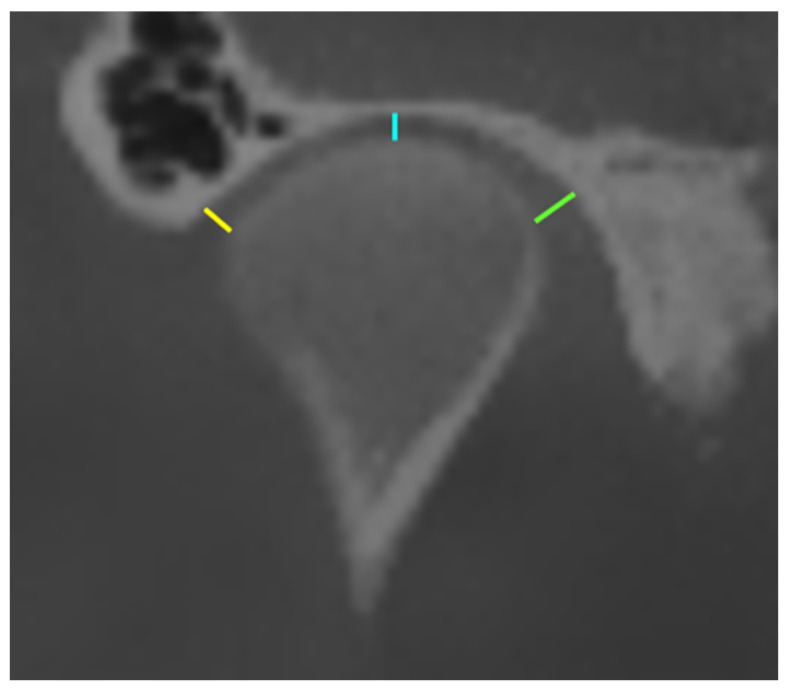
Coronal CBCT image demonstrating DJS (yellow line), CJS (blue line), and MJS (green line) measurements.
